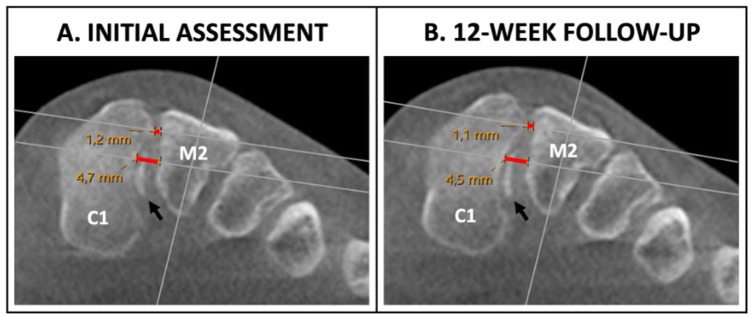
(A) Initial weightbearing computed tomography assessment of a left-sided Lisfranc injury with corresponding measurements of the dorsal Lisfranc ligament (DLL) and interosseous Lisfranc ligament (ILL) distance noted in red (DLL 1.2 mm, ILL 4.7 mm). Notice the presence of an avulsion fracture (black arrow) originating from the ILL inside the C1-M2 junction. After comparing results with the healthy foot, the injury was determined as stable. (B) Twelve-week follow-up evaluation showing preserved C1-M2 stability (DLL 1.1 mm, ILL 4.5 mm). C1 = medial cuneiform, M2 = second metatarsal. [See online article for color figure.]
